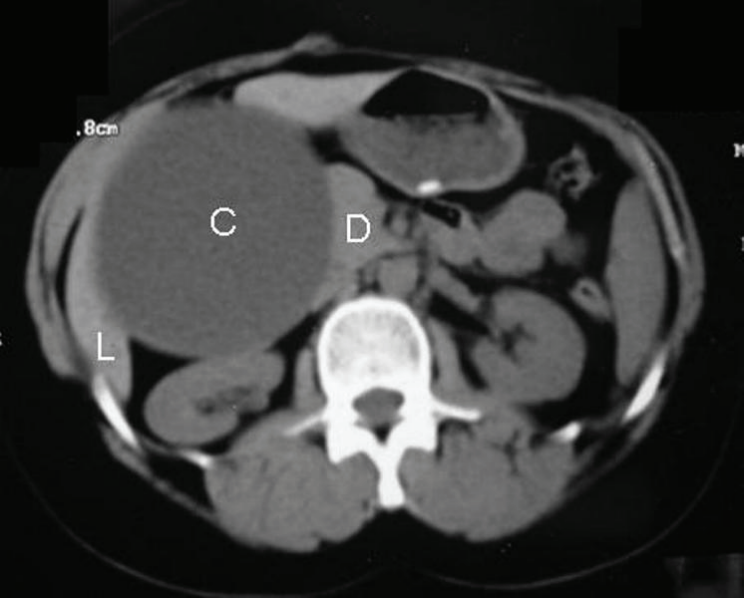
Computed tomography of the abdomen showing a large cystic lesion (C) abutting the Liver (L) and the Duodenum (D).
